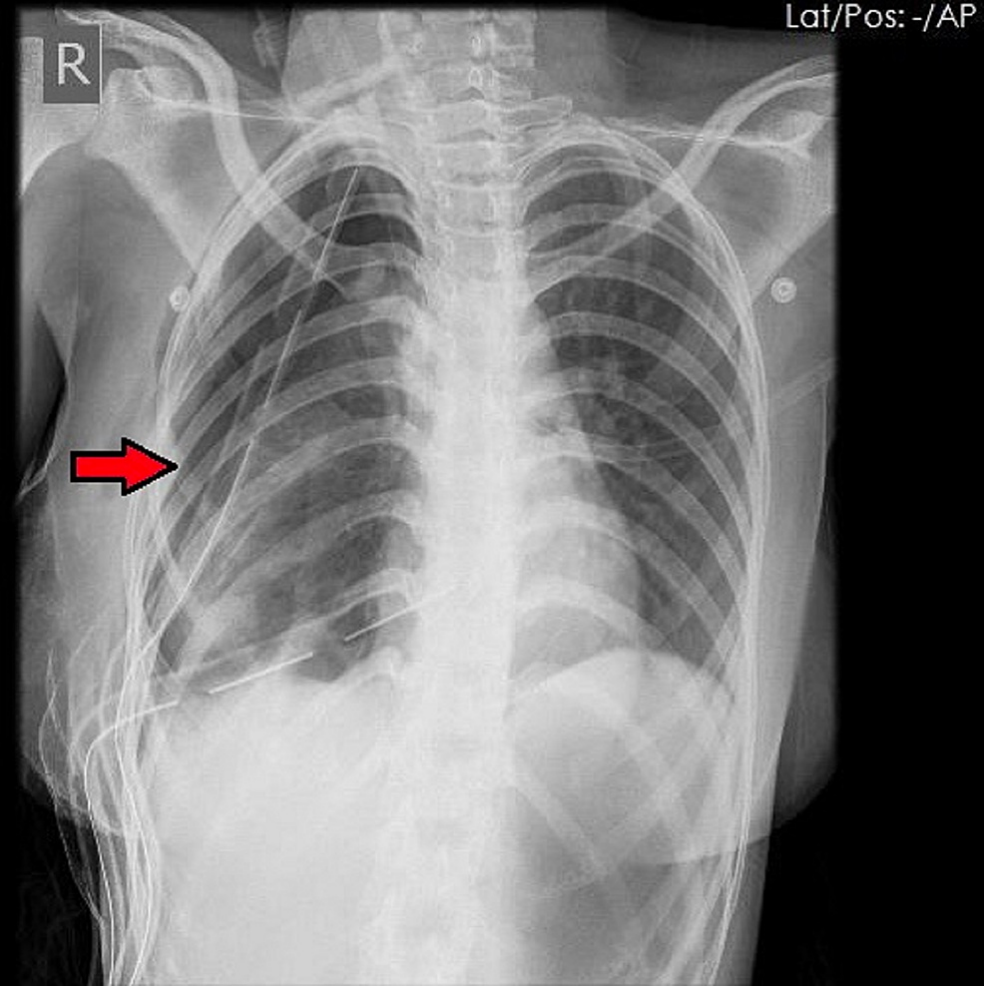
Postoperative chest x-ray showing right lung collapse
